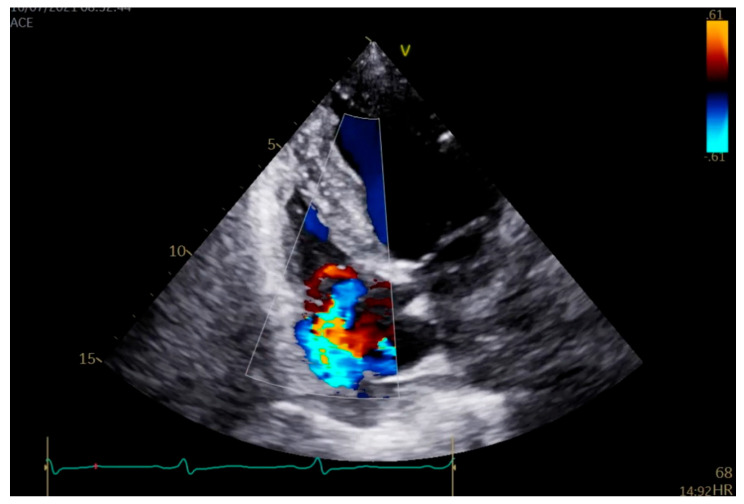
Transthoracic image (four chamber view). Color Doppler showing massive tricuspid regurgitation caused by the infective endocarditis of the tricuspid valve.
